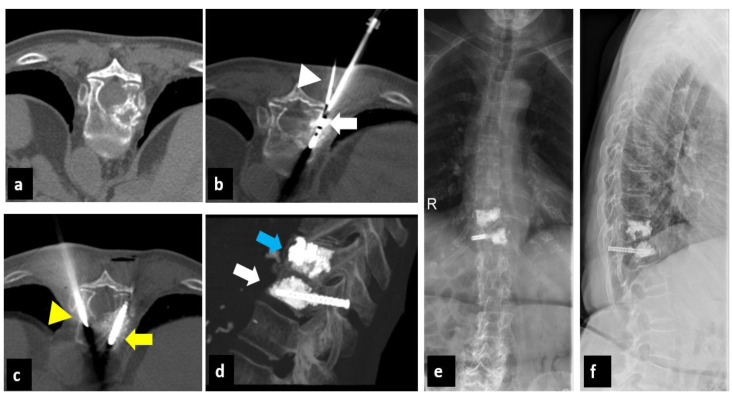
A 84-year-old woman with history of breast cancer, treated with simple vertebroplasty for pathologic D11 vertebral fracture 3 years earlier. (a): Axial CT scan shows osteolytic metastasis of vertebral body D12 involving the right pedicle. (b): Axial CT shows placement of the thermocouple (white arrowhead) in the right anterior epidural space and transpedicular placement of the MW antenna (white arrow) in the D12 vertebral body for thermal ablation. (c): Axial CT shows the placement of the right screw (yellow arrow) and contralateral advancement of the vertebroplasty cannula (yellow arrowhead). (d): Postoperative sagittal CT with Maximum Intensity Projection (MIP) reconstruction after microwave ablation of D12 vertebra combined with pedicle screw fixation followed by vertebroplasty (white arrow). Vertebroplasty of D11 performed 3 years earlier (blue arrow). (e,f): Radiograph of the thoracolumbar spine 3 months after surgery, in antero-posterior and latero-lateral projection, after microwave ablation of the D12 vertebra combined with pedicle screw fixation followed by vertebroplasty (MASFVA).
